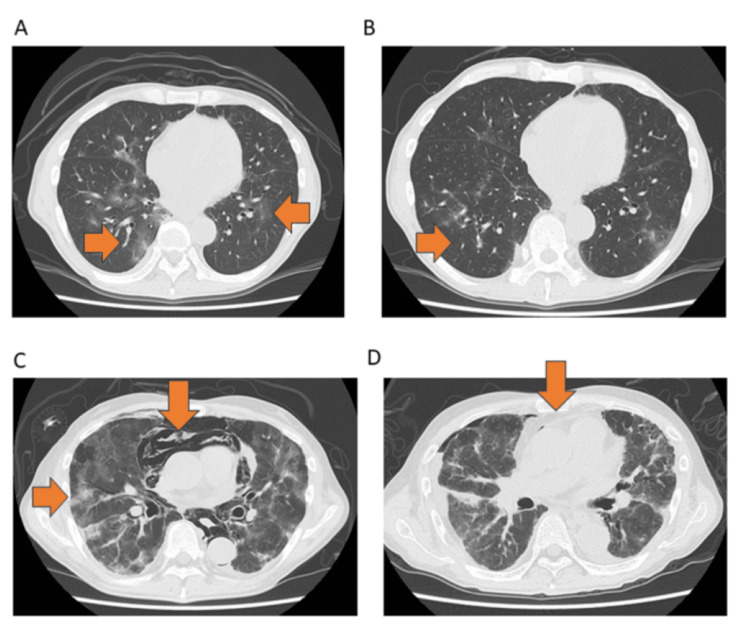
CT images of the patient. (A) Chest CT performed on day 126 after PBSCT revealed bilateral diffuse ground-glass opacities with infiltrative shadows. The arrows indicate the areas of diffuse ground-glass opacities with infiltrative shadows. (B) Chest CT performed on day 150 after PBSCT showed improvement in COP. The arrow indicates the resolution of the ground-glass opacities and infiltrative shadows. (C) Chest CT performed on day 261 after PBSCT revealed bilateral diffuse ground-glass opacities with infiltrative shadows and confirmed the presence of pneumomediastinum. The arrows indicate the areas of diffuse ground-glass opacities with infiltrative shadows and pneumomediastinum. (D) Chest CT performed on day 342 after PBSCT, corresponding to 77 days after the initiation of ruxolitinib treatment, showed improvement in pneumomediastinum. The arrow indicates the resolution of the pneumomediastinum. CT, computed tomography; PBSCT, peripheral blood stem cell transplantation; COP, cryptogenic organizing pneumonia
